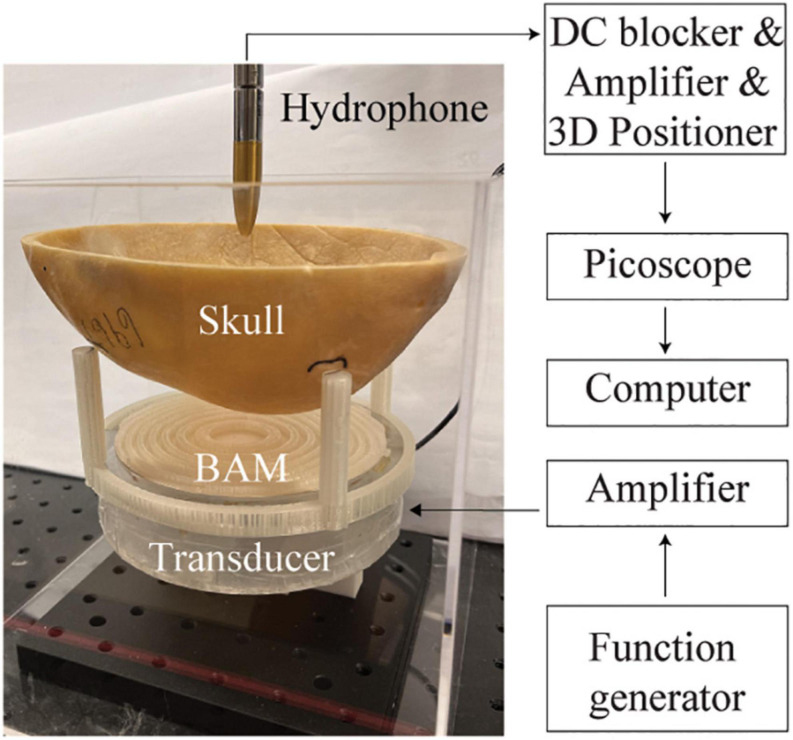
Experimental setup for validating the designed BAM coupled with a plat ultrasound transducer.
